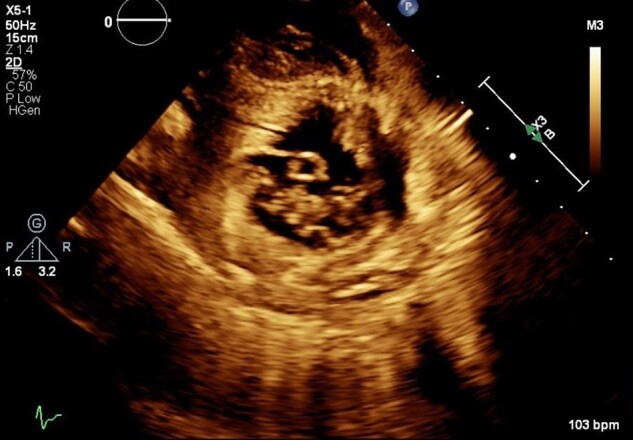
Transthoracic echocardiogram. Basal parasternal short axis at the mitral valve view showing pedunculated finger-like, cystic mass (2×0.72 cm) protruding into the cavity of the left ventricle. Cross sectional view of mass with echo lucent core.
